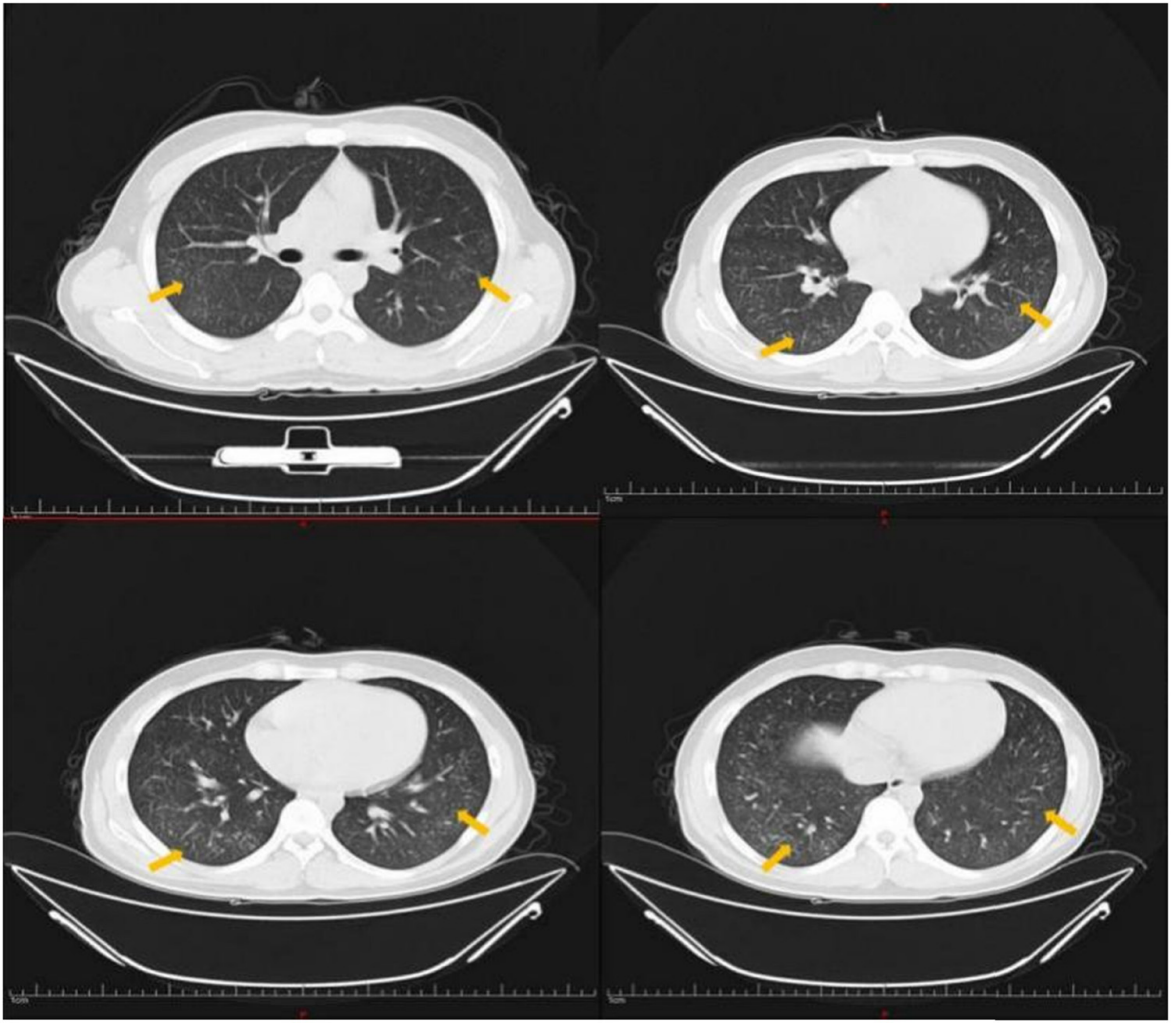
Diffuse miliary nodules in both lungs were observed on chest CT.
